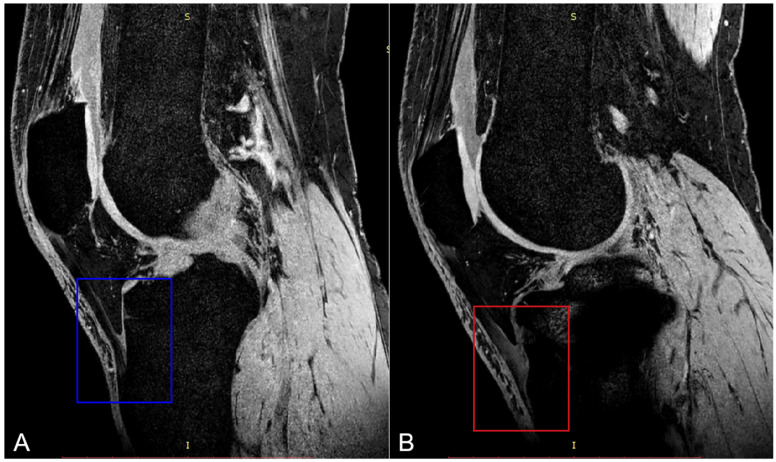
One of 15 patients’ knee MR images was randomly selected to display the typical changes happening in distal PT bundles at 6 months after OWHTO ((A) preoperative MRI; (B) postoperative MRI).
